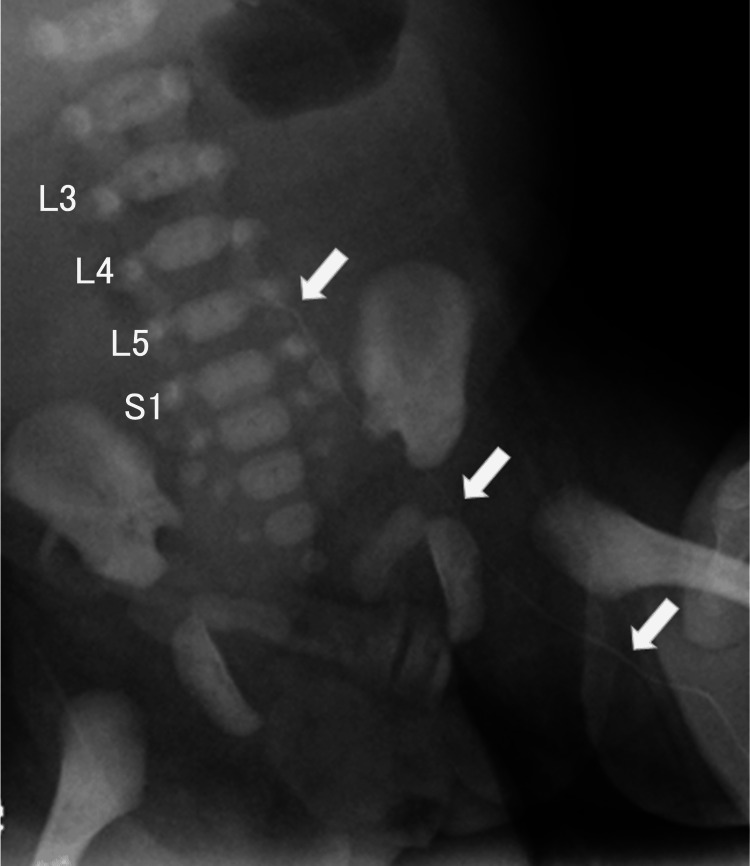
Chest-abdominal radiograph showing an abnormal course of the catheter The catheter (arrow) ascends along the left side of the vertebral column and curves medially at the level of the fifth lumbar vertebra. Vertebral levels (L3, L4, L5, and S1) are labeled on this image.
